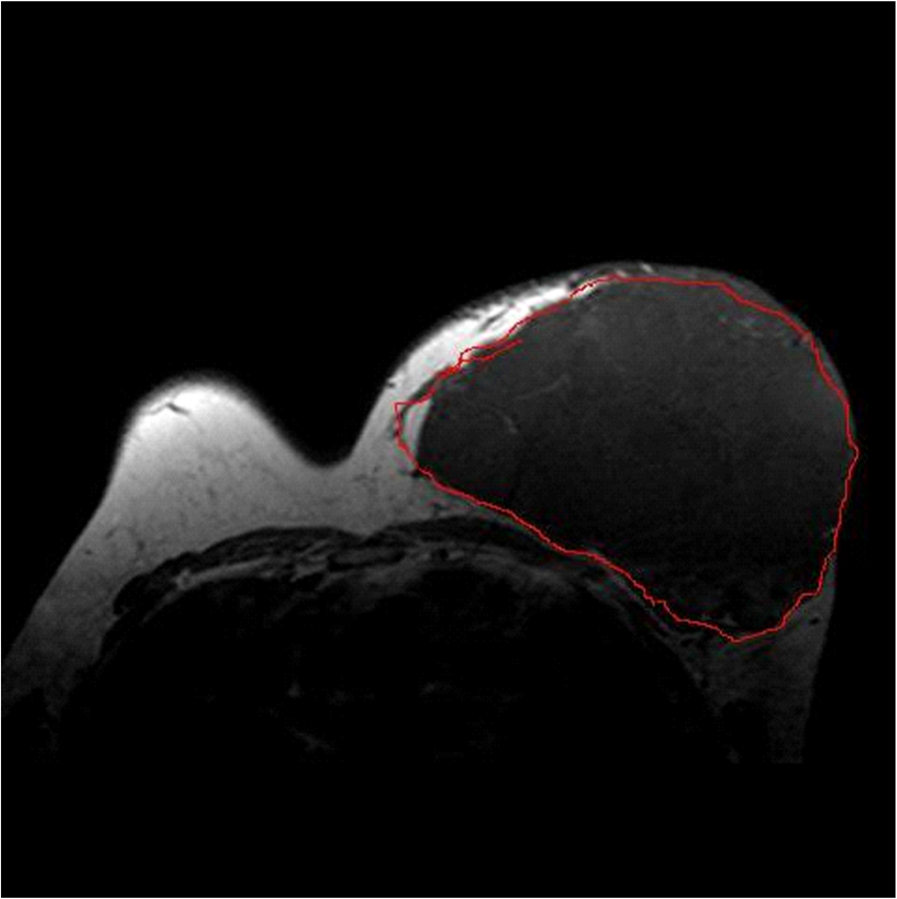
The presence or absence of breast tumor and the diameter of the tumor were determined by preoperative MRI
